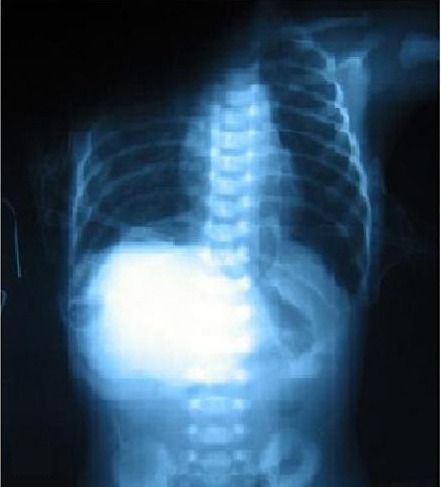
Figure 3: Postoperative chest radiograph.
